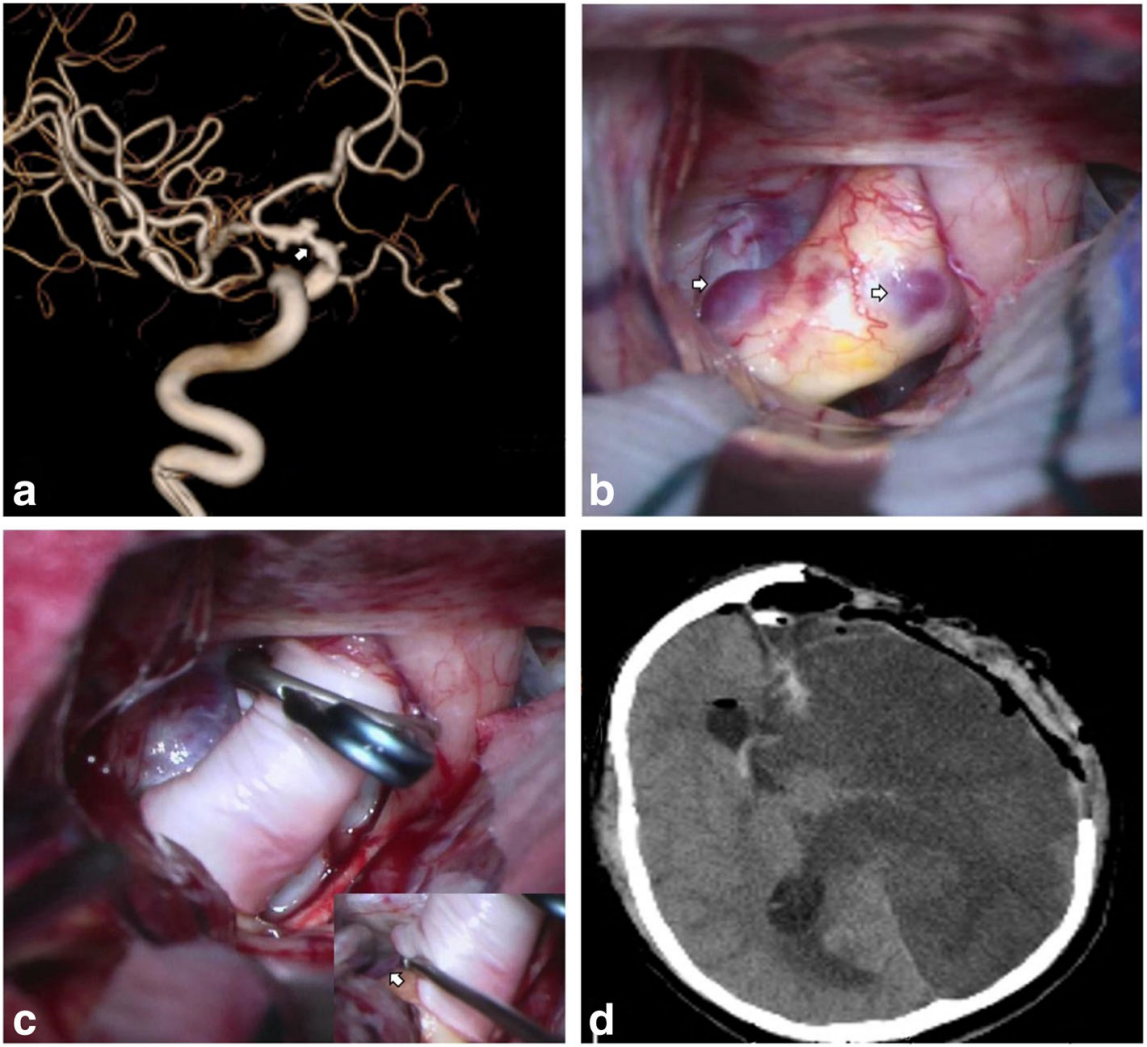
Case 8. a Preoperative 3D DSA demonstrated two neighboring ICA BLAs and notably stenotic lumen (white arrow). b Intraoperative findings showed the macroscopic features of both BLAs (white arrow) and entirely sclerotic parent wall. c Both BLAs were obliterated and the inset showed patency of the left anterior choroidal artery (white arrow). d Postoperative CT revealed left hemispheric infarct
